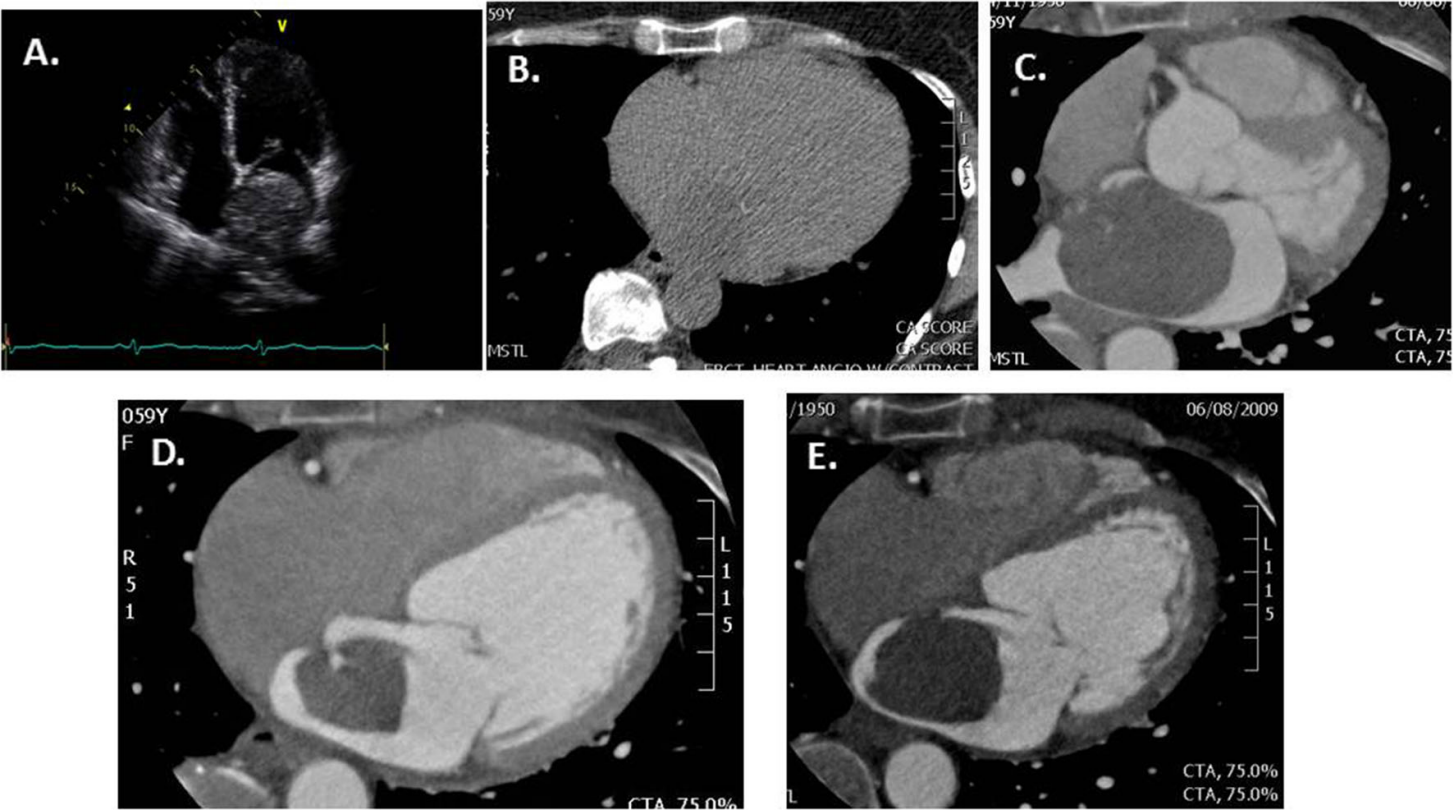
Atrial myxoma. (a) Apical four-chamber view on transthoracic echocardiogram demonstrating a large mass occupying the majority of the left atrial cavity. (b) Non-contrast axial image faintly demonstrating the large left atrial mass. (c-e) Cardiac CT angiography images demonstrating a large, low attenuating mass with a small stalk attached to the fossa ovale, consistent with a large left atrial myxoma. Note the patchy, non-homogenous contrast enhancement
